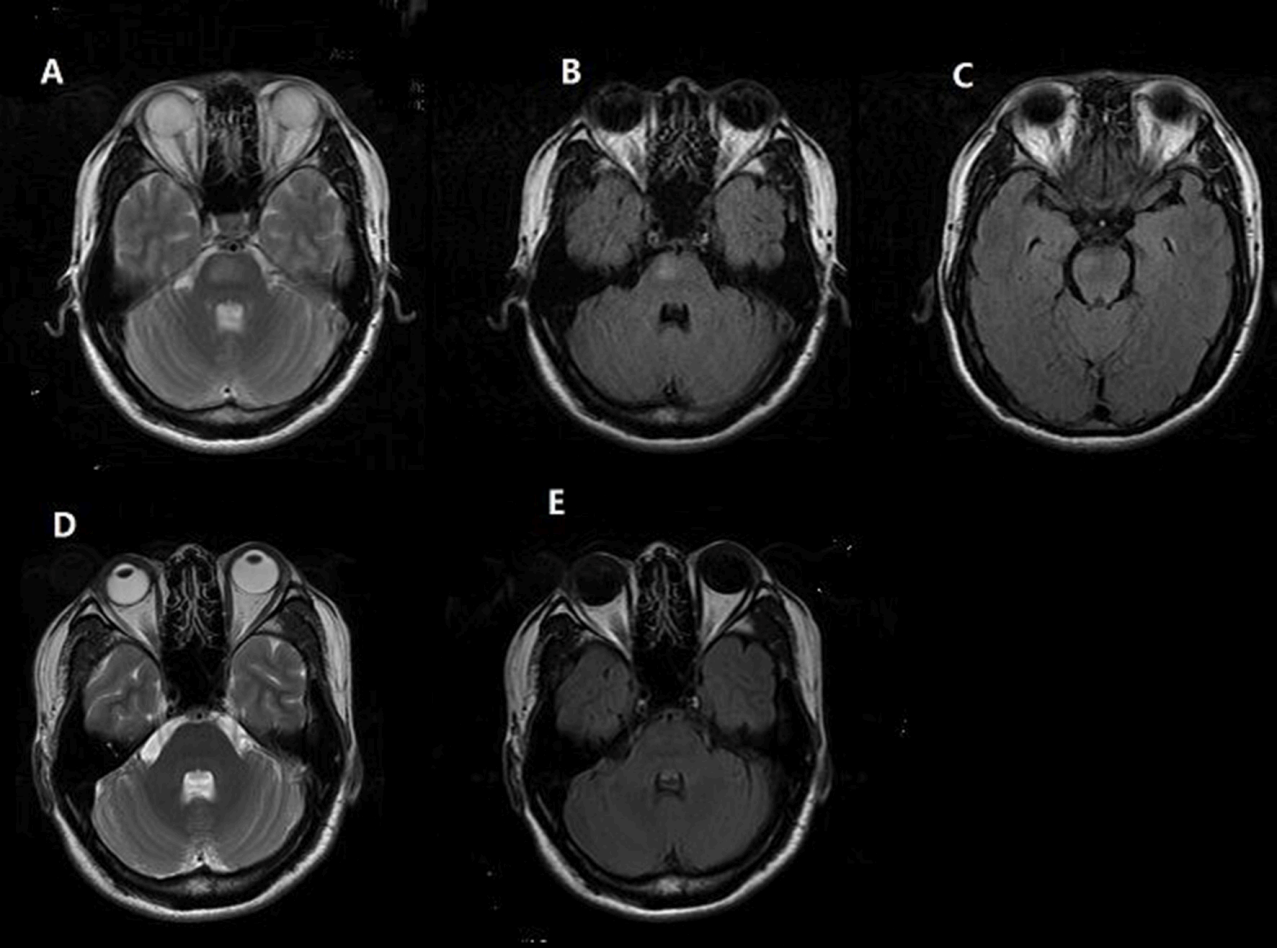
Brain MRI finding. Patient 1, (A,B) Axial T2-weighted and fluid attenuated inversion recovery (FLAIR) MR image reveals hyperintense lesion in pons. (C) No abnormal signal in the parietal and occipital lobes was found. (D,E) Follow-up MRI at 1 month shows complete resolution of the hyperintensity in the brainstem.
